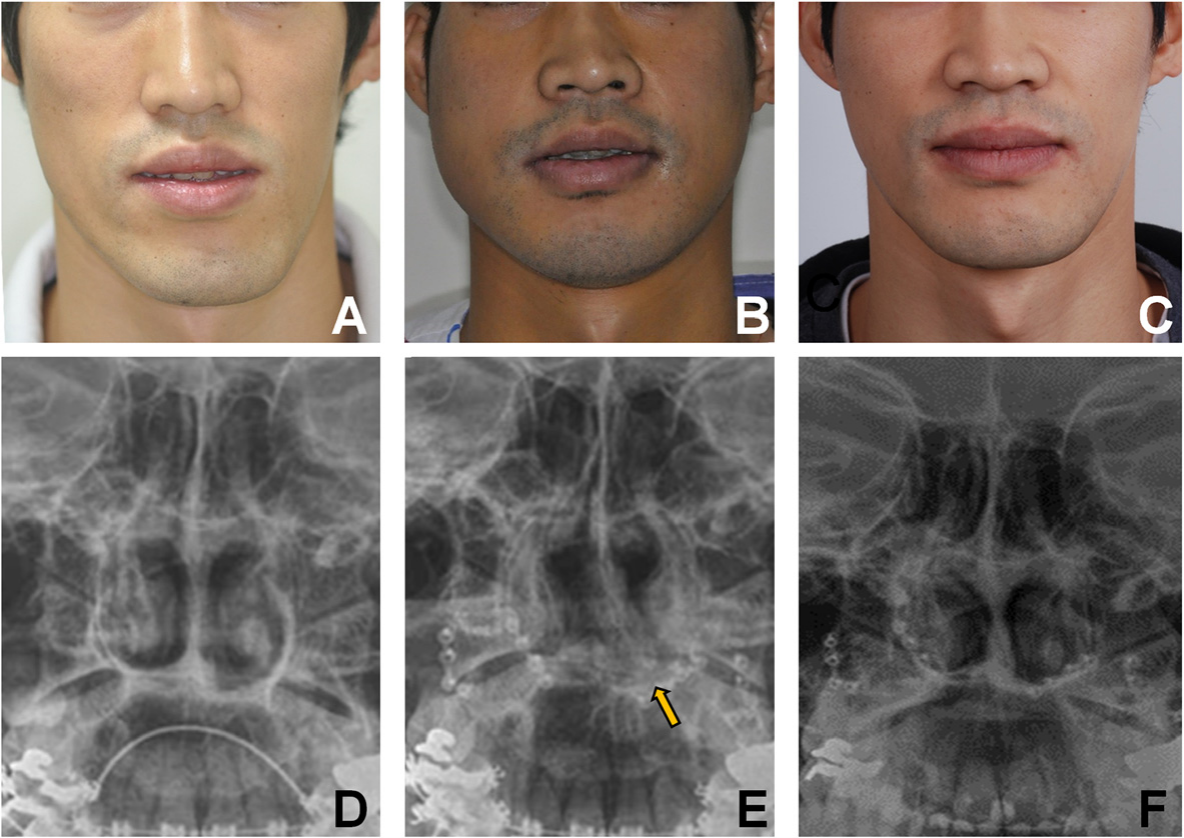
Clinical and radiographic findings of the case 3 patient before Le Fort I osteotomy (a, d), immediate after Le Fort I osteotomy (b, e), The *arrow* indicates the caudal part of the septal cartilage (e). Sepal deviation was successfully resolved after the corrective surgery (c, f)
